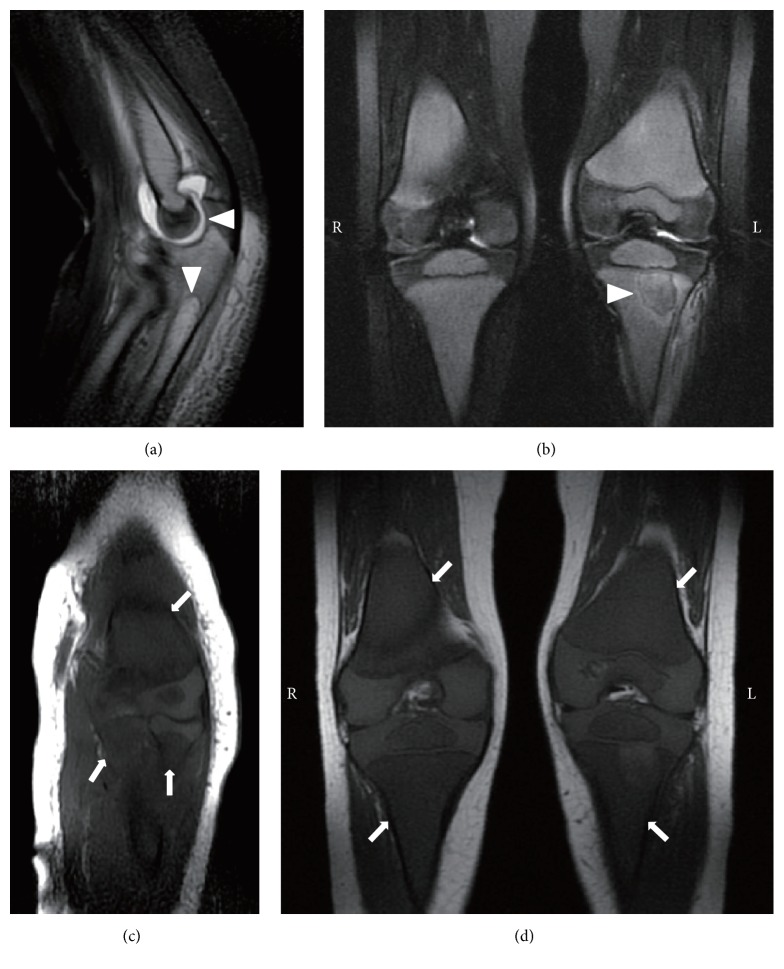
MRI findings in case 4. At 6 days after the disease onset, (a) T2-weighted image shows accumulation of synovial fluid and high signal intensity in the ulnar bone marrow (arrowheads) and (c) T1-weighted images depict diffuse low signal intensity in the left humeral, radial, and ulnar bone marrow (arrows). At 18 days after the onset, (b) T2-weighted image reveals local high signal intensity in the left tibial bone marrow (arrowhead) and (d) T1-weighted image depicts diffuse low signal intensity in the bilateral femoral and tibial bone marrow (arrows).
